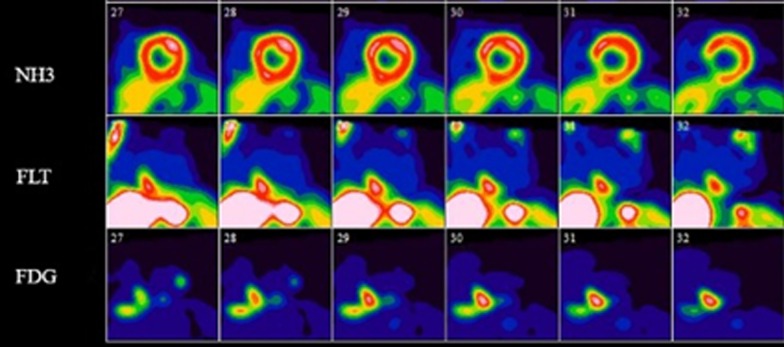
Selected short axis images of cardiac perfusion PET (top), FLT (middle), and FDG (bottom) studies in short, vertical, and horizontal long axes in a single subject with CS. The findings were consistent with active CS on both the FLT and FDG studies. The images show the relationship between an area of scarring in the inferior aspect of the ventricular septum which overlaps an area of concordant uptake on both FDG and FLT
